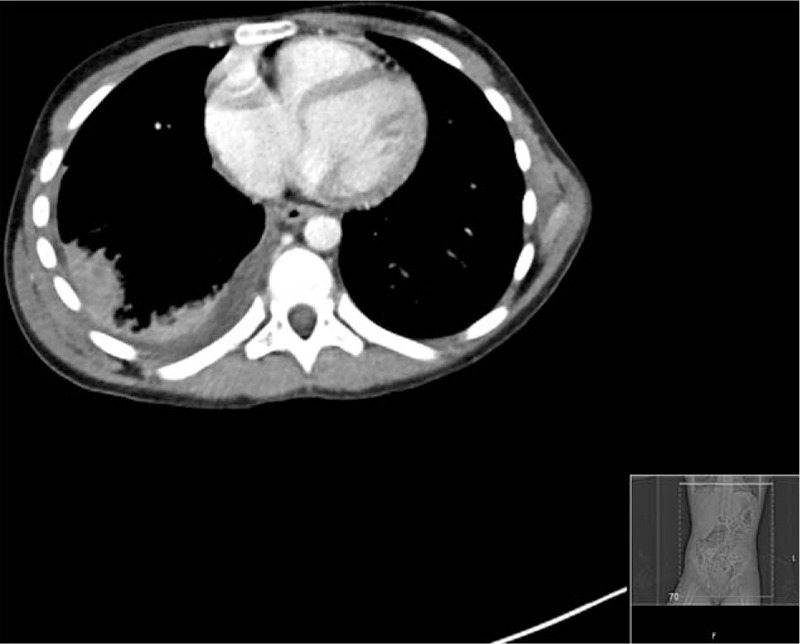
Transverse slice of computed tomography scan of abdomen and pelvis showing right lower lobe consolidation.
